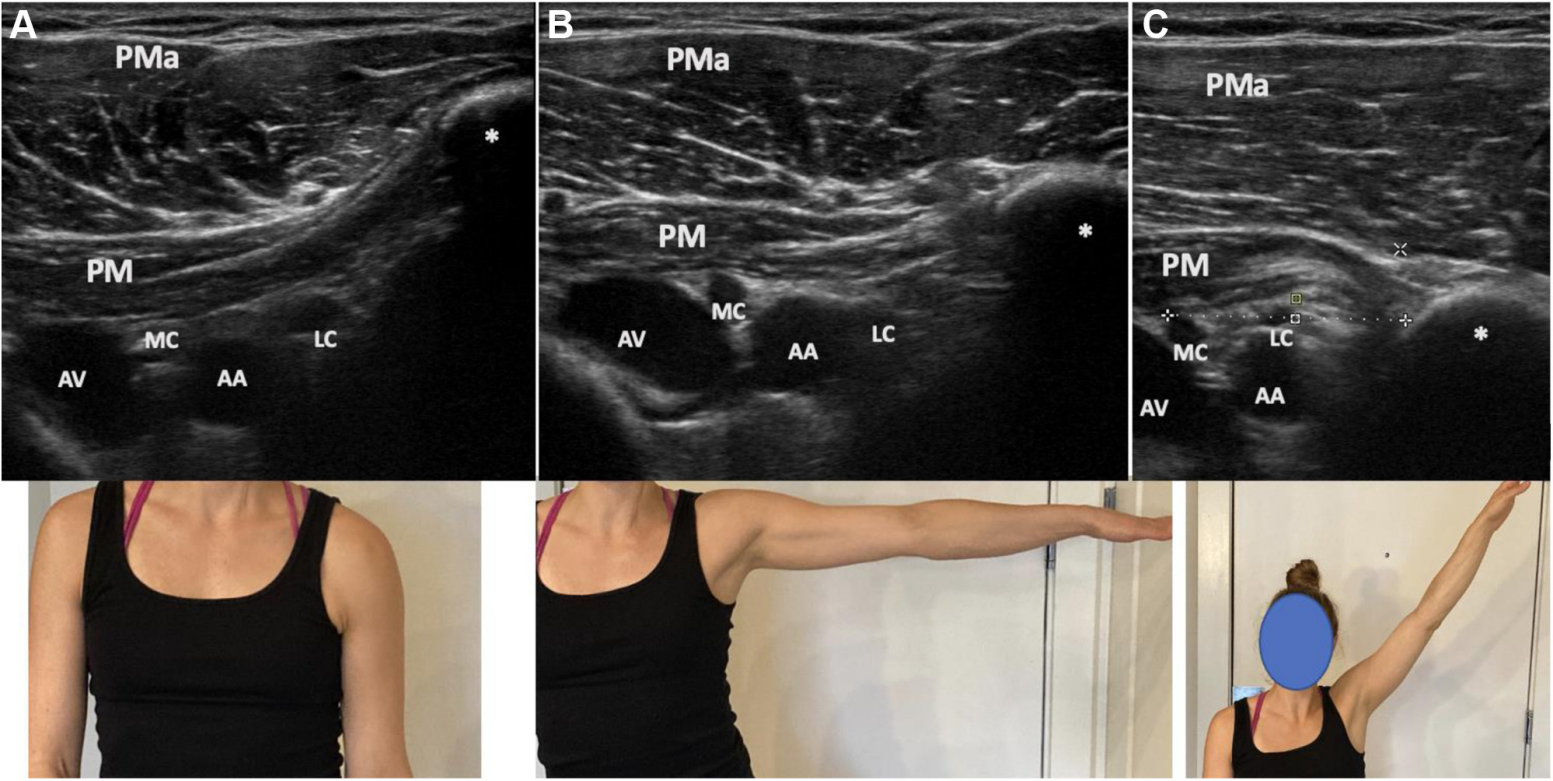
Dynamic ultrasound evaluation of the left pectoralis minor during active arm abduction in the plane of the body, in a patient with symptoms of neurogenic thoracic outlet. Note the indentation of the posterior aspect of the pectoralis minor during progressive abduction, as well as the proximity of the axillary vessels and cords compared to the patient in Figure 6, a consequence of decreased volume in the retropectoralis minor space. Arm at side (A), arm in abduction (B), and maximal abduction (C). *AA*, axillary artery; *AV*, axillary vein; *LC*, lateral cord; *MC*, medial cord; *PM*, pectoralis minor; *PMa*, pectoralis major; ∗ indicates coracoid.
